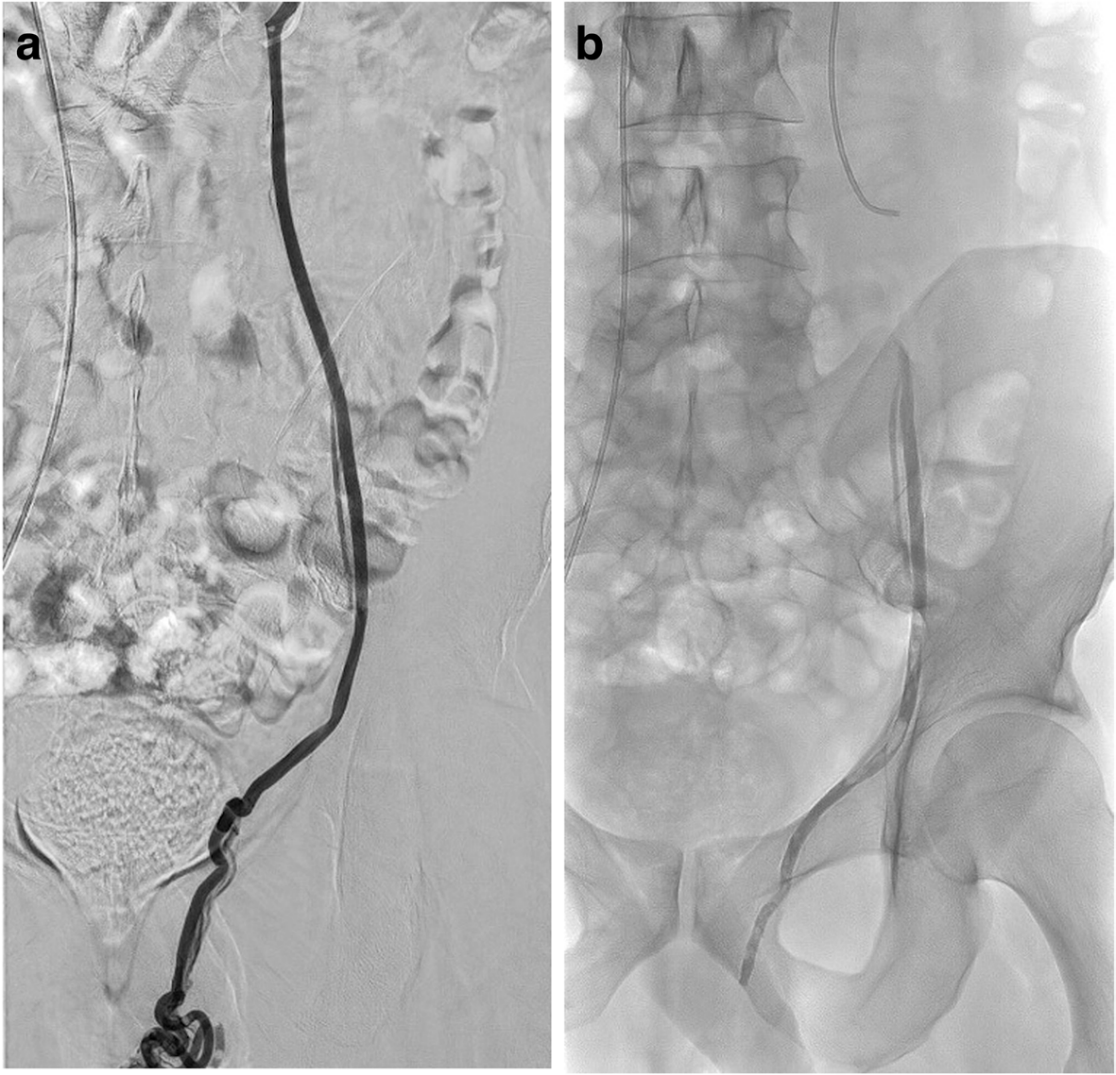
Left varicocele responsible for pain in a 28-year-old man. a Left spermatic venography through a 5-French Cobra catheter by the transvenous femoral approach showing two venous channels. b Plain radiograph after left spermatic vein embolisation using AHCA-MagicGlue®/Lipiodol® in a 1:1 ratio: note the glue cast along the main left spermatic vein with reflux into the second, smaller channel producing complete occlusion of all collaterals. The left testicle pain resolved within 1 month and the follow-up sonogram showed no reflux into the pampiniform plexus
